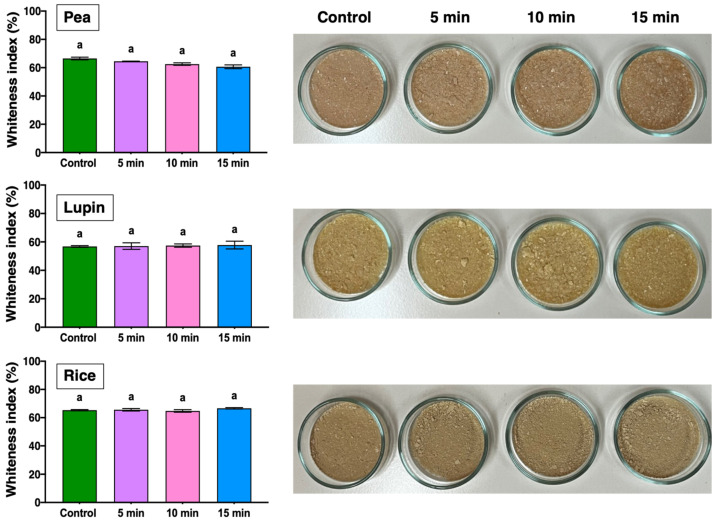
Whiteness index of different freeze-dried plant proteins treated at different times of ultrasound. Different letters indicate significant differences (*p <* 0.05) between means for US treatment times.
